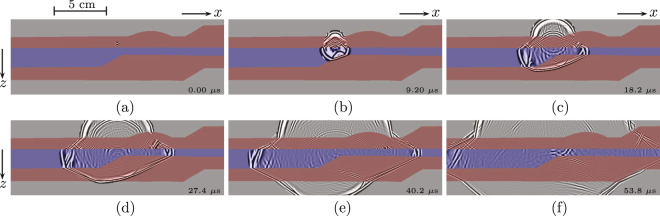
Virtual response *G*(r, s, *t*) + *G*(r, s,−*t*), retrieved from the single-sided ultrasonic reflection response *G*(x′, x, *t*) of the physical model in Fig. 2(a). (a) *t* = 0 *μ*s. (b) *t* = 9.2 *μ*s. (c) *t* = 18.2 *μ*s. (d) *t* = 27.4 *μ*s. (e) *t* = 40.2 *μ*s. (f) *t* = 53.8 *μ*s.
